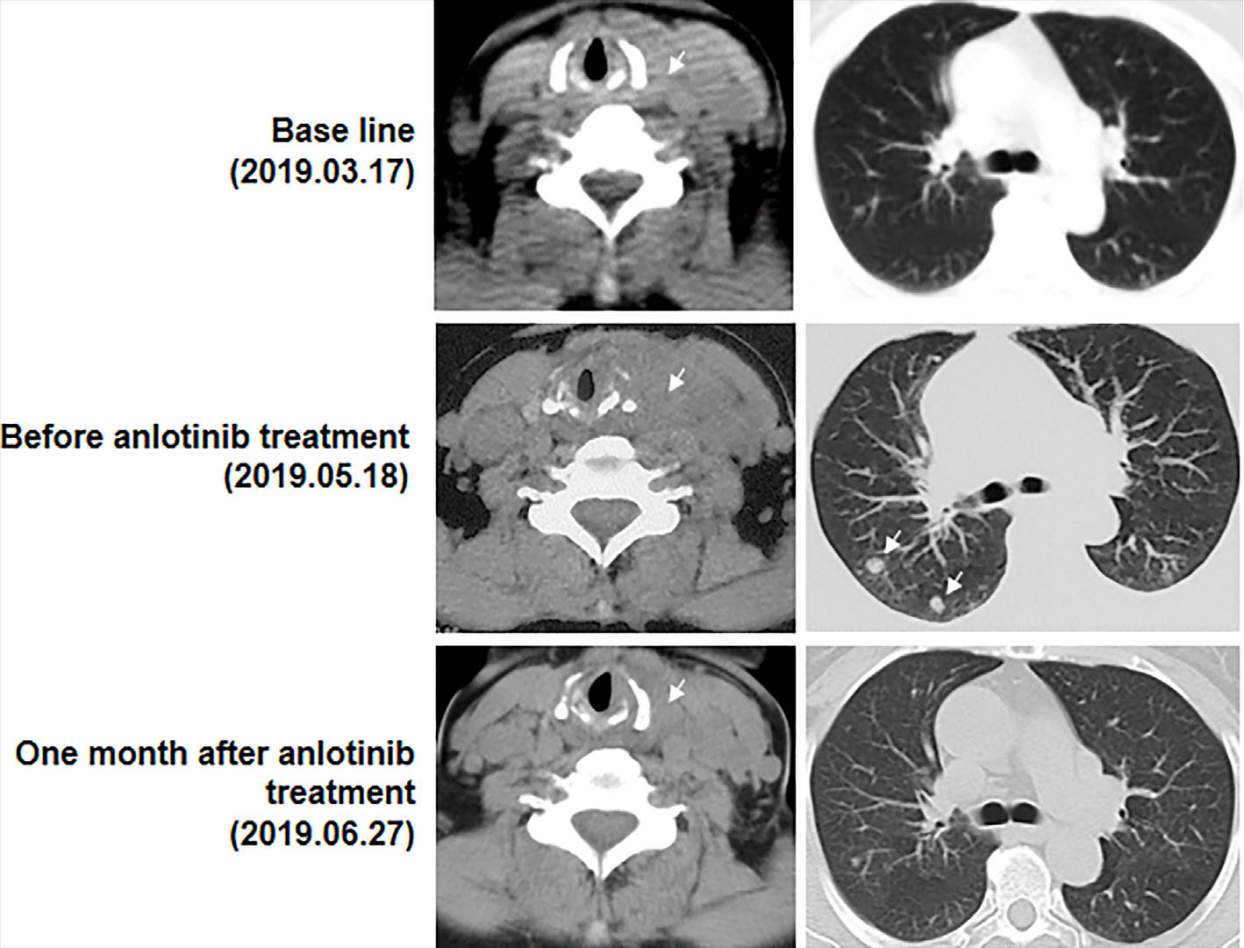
Computed tomography images (CT) of the thyroid and lung before and after anlotinib treatment. Arrows represent the masses. At base line, in addition to brain lesions, there were a mass in the left thyroid and some opaque mottled shadows and pulmonary nodules in the lung. Then the patient experienced brain surgery. Before anlotinib treatment, a huge mass in the left thyroid and two obvious pulmonary nodules was found. One month after anlotinib treatment, the pulmonary nodules were found to be controlled, thyroid tumor drastically reduced and tracheal compression relieved.
